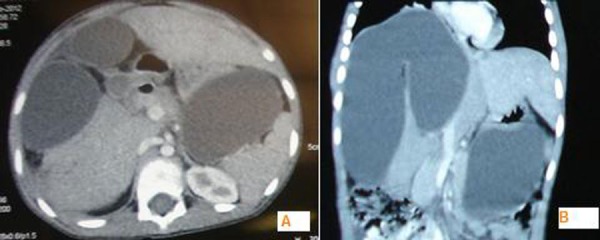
Figure 2: A-Axial section of CT scan showing cystic lesion in the right lobe of the liver with well defined septa of liver parenchyma within it. The lesion in lesser sac is also appreciated B- Same in coronal section.
